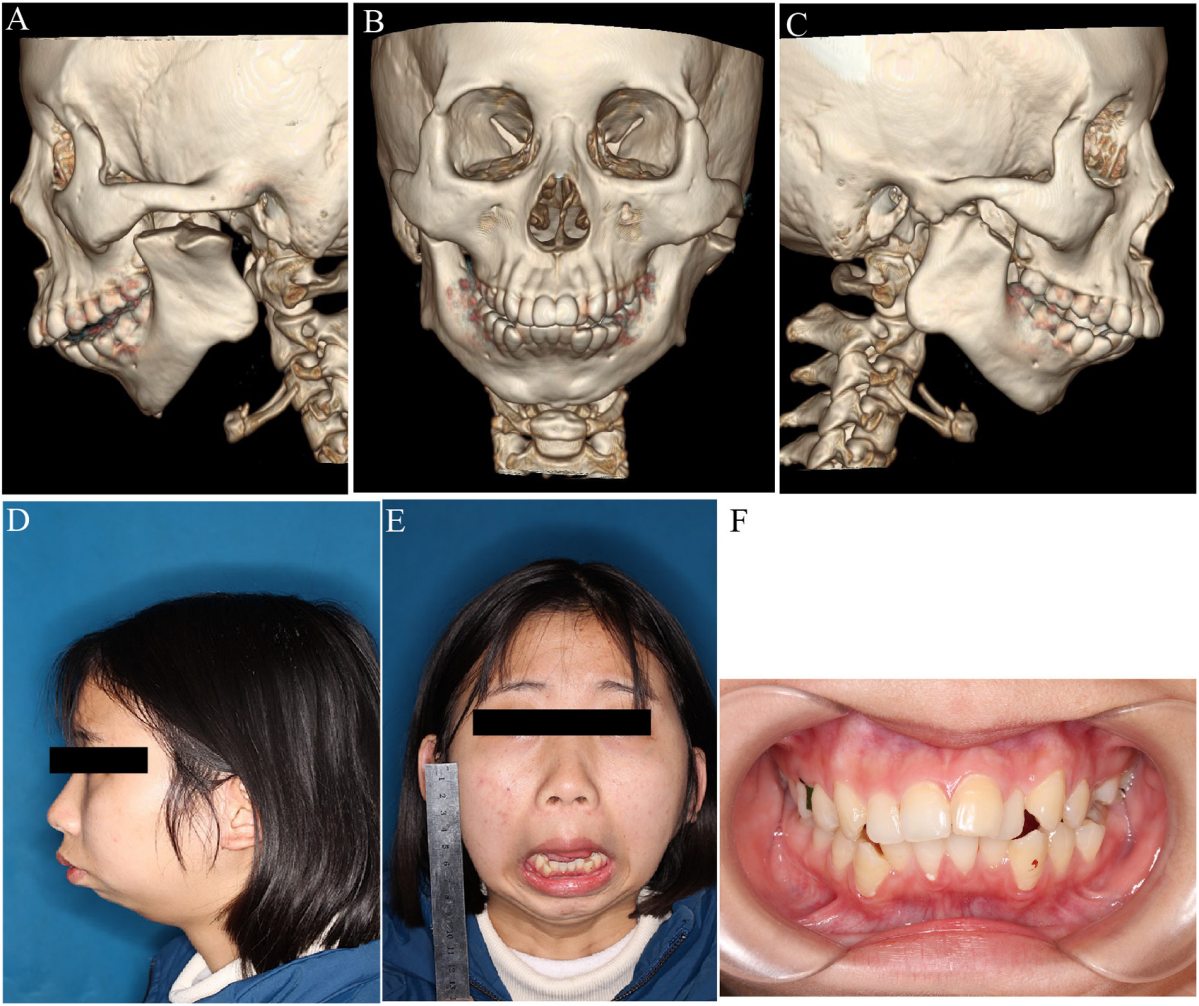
Postoperative evaluation of mandibular morphology and function. (A–C) 3D CT reconstructions after arthroplasty showing structural modifications; (D,E) Preoperative lateral and frontal facial views; postoperative maximum interincisal opening (MIO) measured at 3.5 mm; (F) Intraoral image showing occlusal relationship postoperatively.
